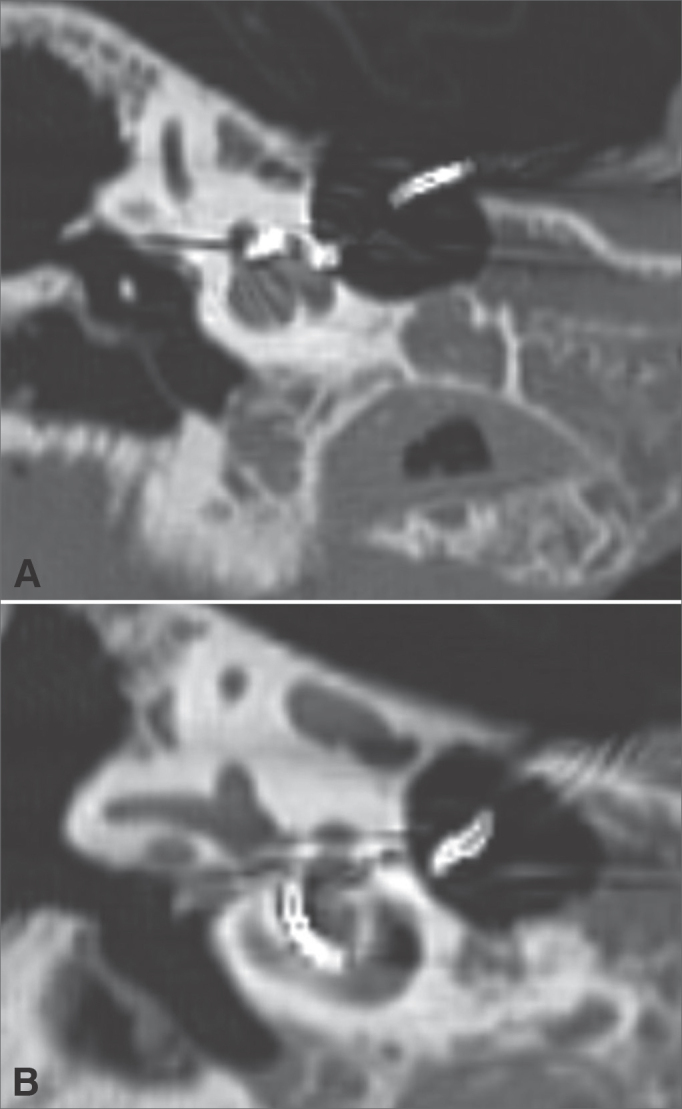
A-B: Right temporal bone high-resolution CT scan. Coronal view, bone window, showing the placement of the array from the basal (A) to the apical (B) turn of the cochlea.
